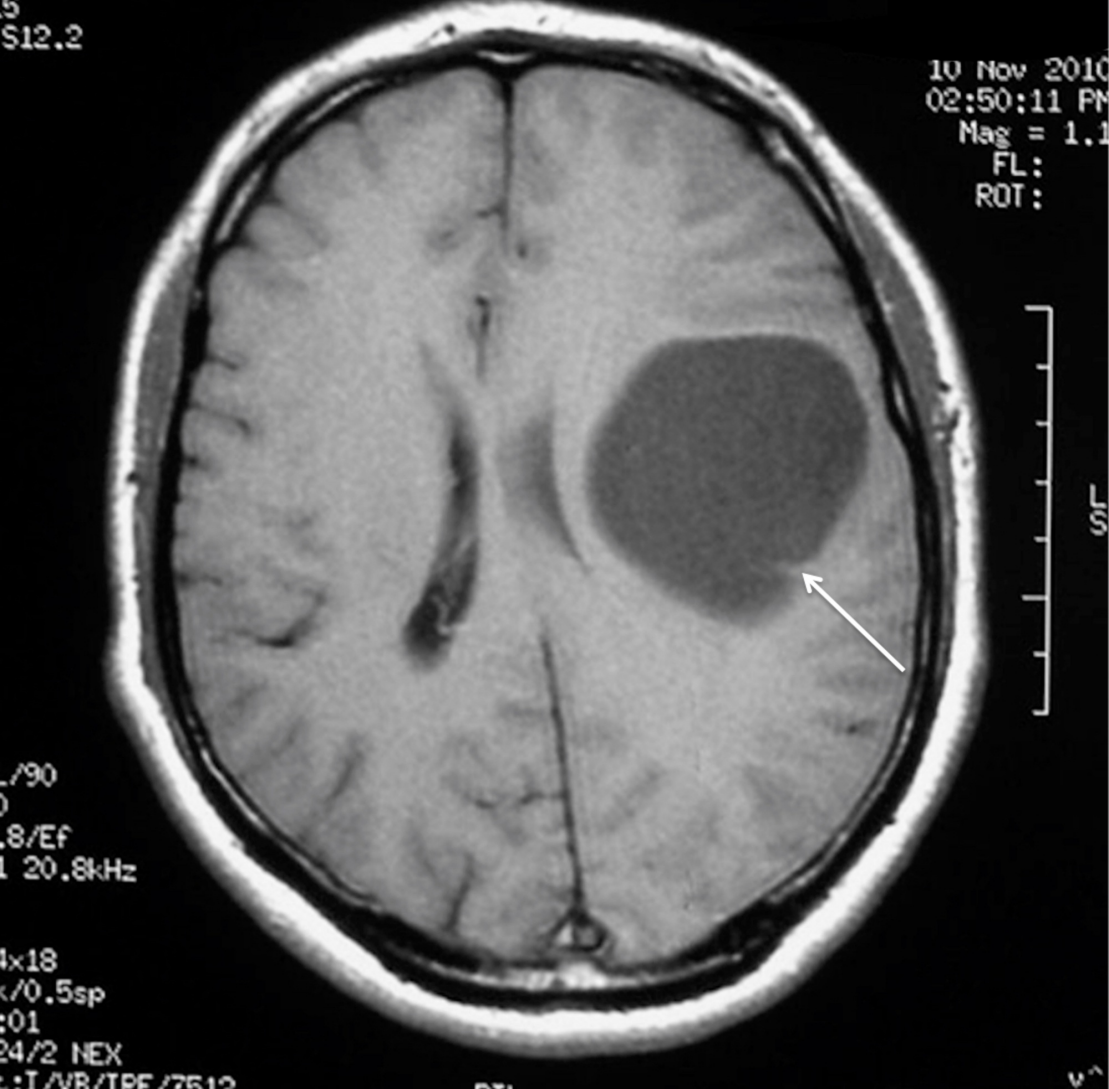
Preoperative MRI without enhancement demonstrating left frontal cystic tumour with septum in the occipital part on the T1WI axial view (arrow). MRI: Magnetic resonance imaging; T1WI: T1-weighted images.
